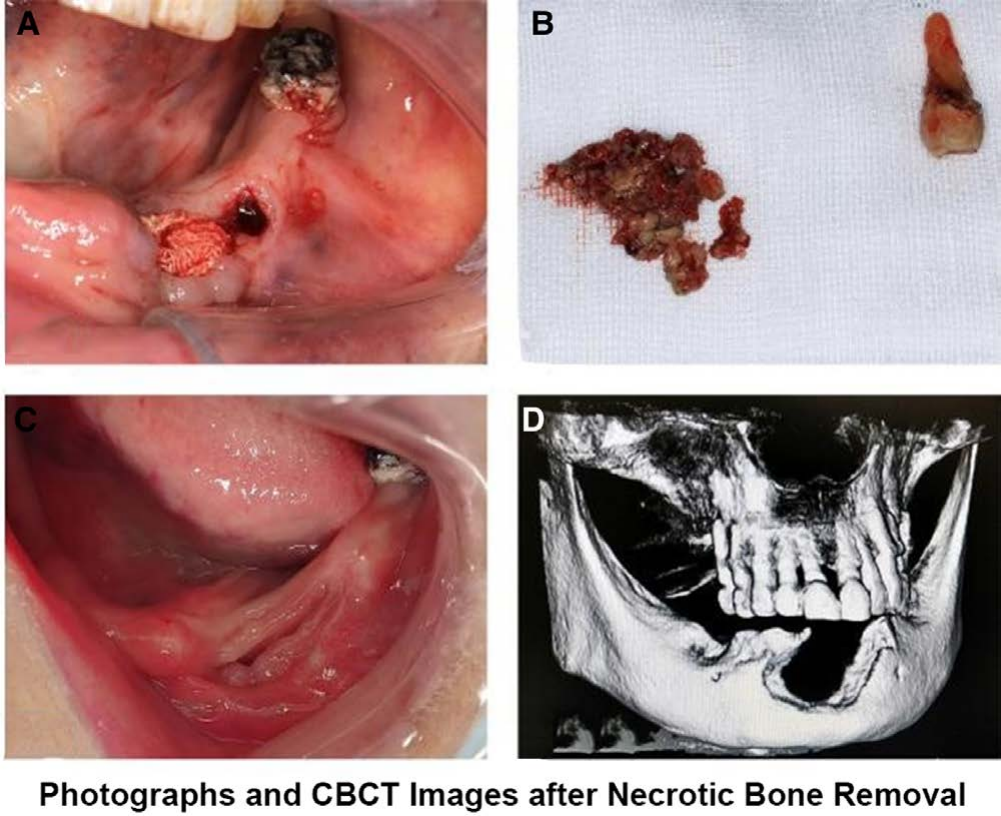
Photographs and CBCT images after necrotic bone removal. (A) Intraoral photographs showing the surgical site packed with iodine-soaked gauze. (B) Digital photographs displayed the excised necrotic bone and the extracted tooth. (C) Intraoral images demonstrated satisfactory surgical wound healing at one month postoperatively, characterized by the absence of evident redness or purulent discharge. (D) Postoperative CBCT imaging showing the absence of necrotic bone in the anterior mandibular region. CBCT = cone-beam computed tomography.
